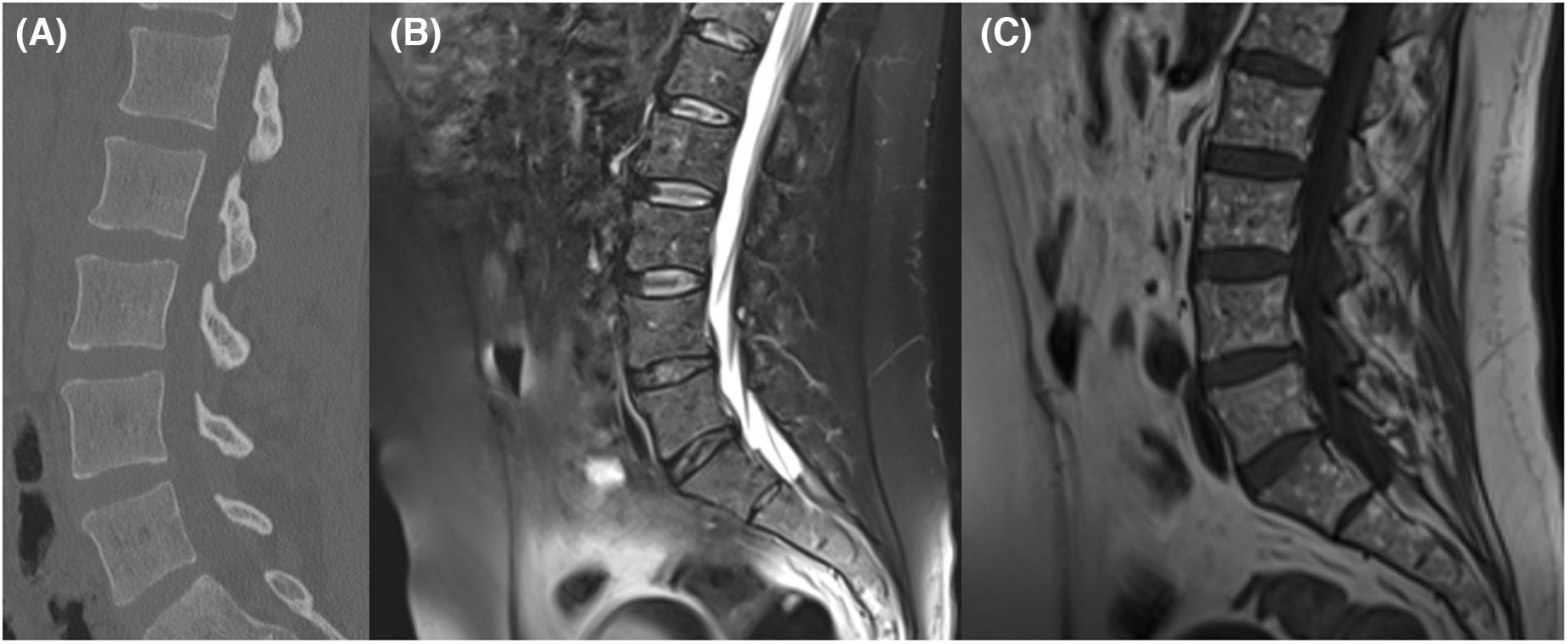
(A) Normal lumbar spine CT in the emergency department; (B) (Sagittal T2 fat saturated) and (C) (Sagittal T1)—lumbar spine MRI with no post‐traumatic changes but with a “salt and pepper” pattern seen on the sagittal T1 sequence.
